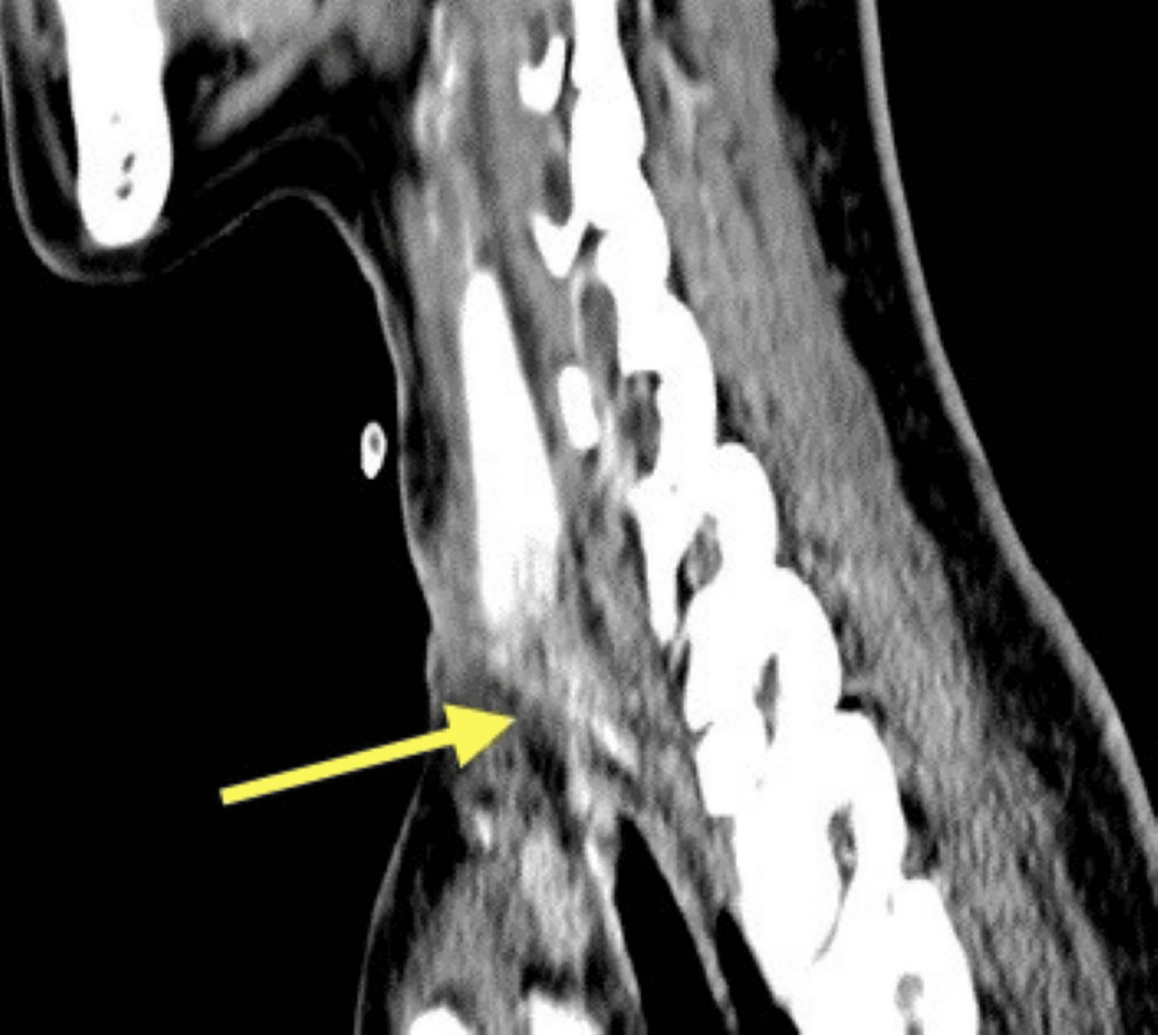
CT sagittal view: the laryngotracheal area was obliterated due to soft tissue edema, the thyroid cartilage was eroded, and the cricoid cartilage was absent (yellow arrow).
